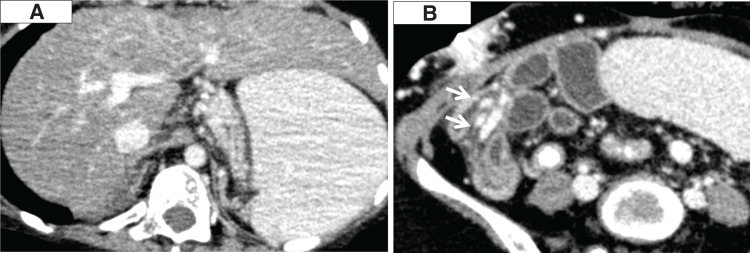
Pre-transplant CT images. (A) Liver cirrhosis and splenomegaly. (B) Prominent collateral circulation (arrows).
